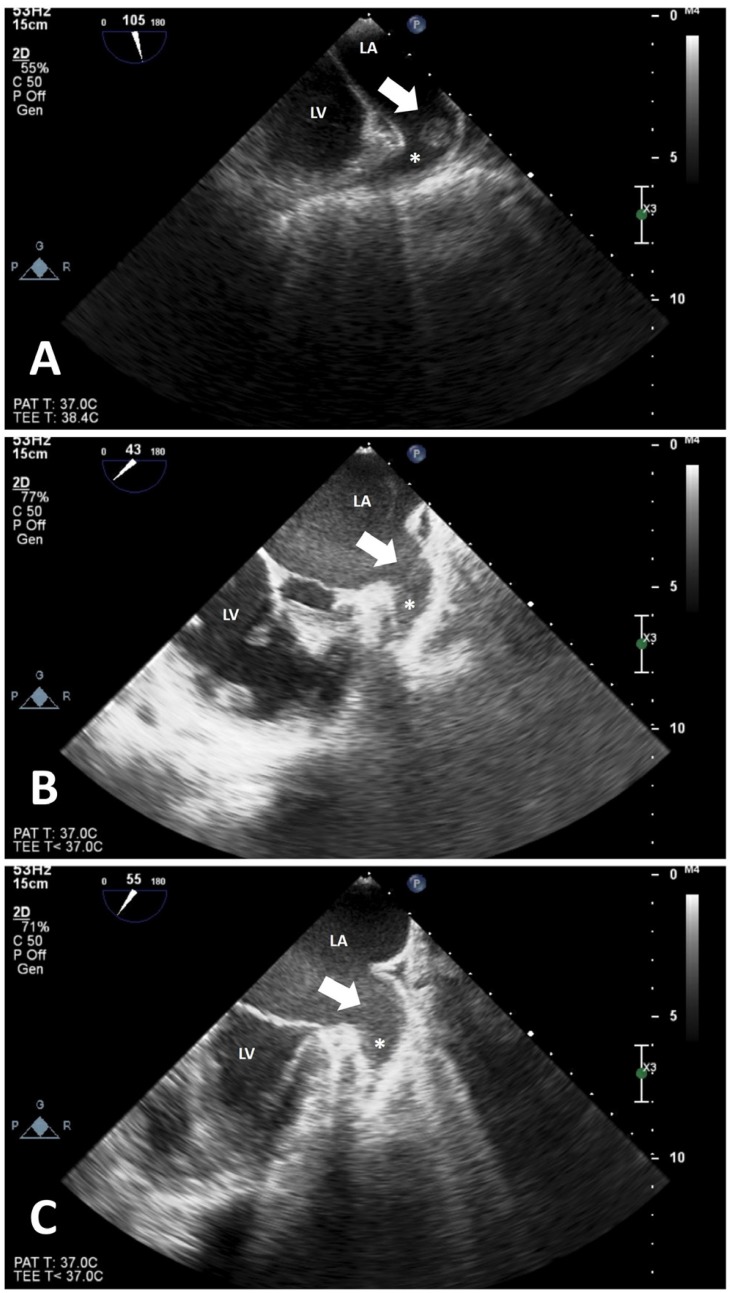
Transesophageal echocardiographic images show the stages of the thrombus by time. The thrombus and the spontaneous echo contrast were completely resolved after the commencement of warfarin.
